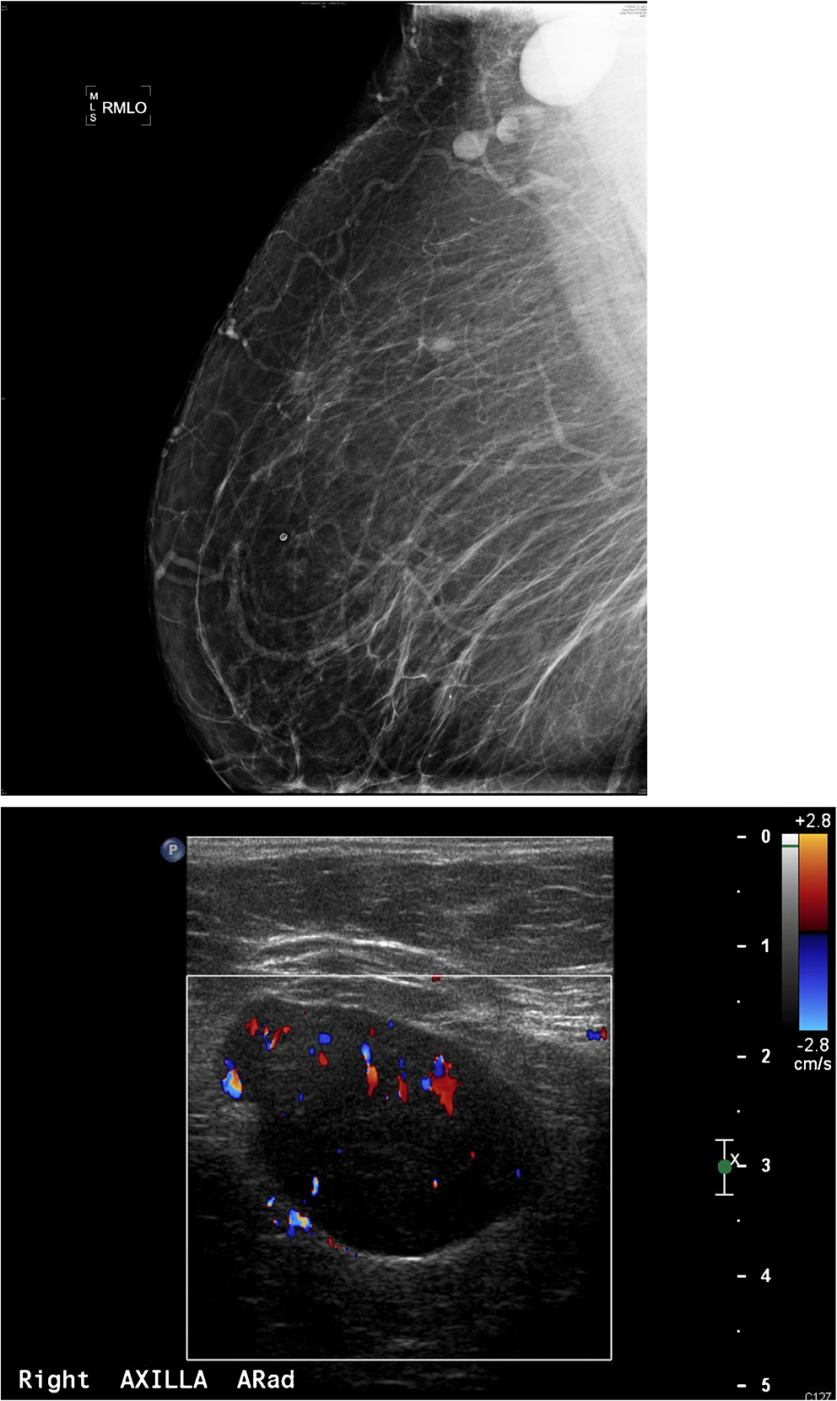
A 55-year-old African American female for screening. a MLO views of the breasts demonstrate a dense enlarged right axillary lymph node and several abnormal-appearing intramammary lymph nodes. Right axillary ultrasound confirms a large fatty replaced lymph node with non-hilar internal vascular flow. This is highly suspicious for malignancy in a patient with breast cancer; however this patient had a negative mammogram and so an ultrasound-guided core biopsy was performed for diagnosis. Final diagnosis: sarcoidosis
